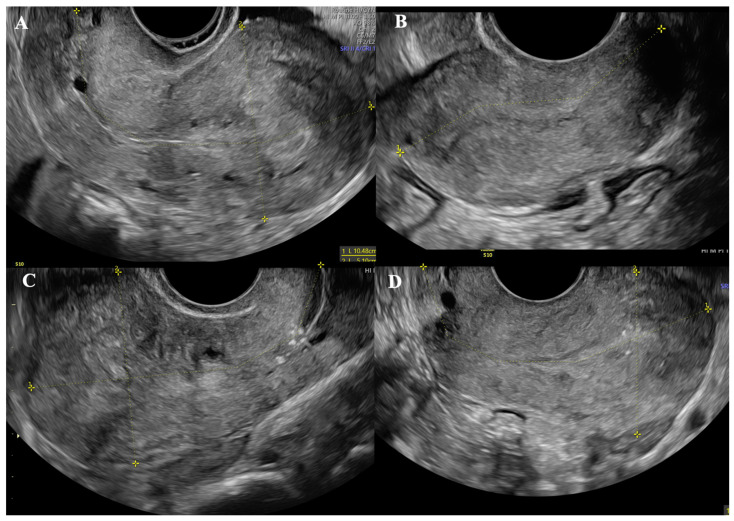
Examples of the main sonographic features of adenomyosis. Myometrial cysts (A), hyperechoic islands (A), subendometrial lines and buds (D), globular appearance of the uterus (A–D), asymmetry of wall thickness (A–C), fan-shaped shadowing (A).
